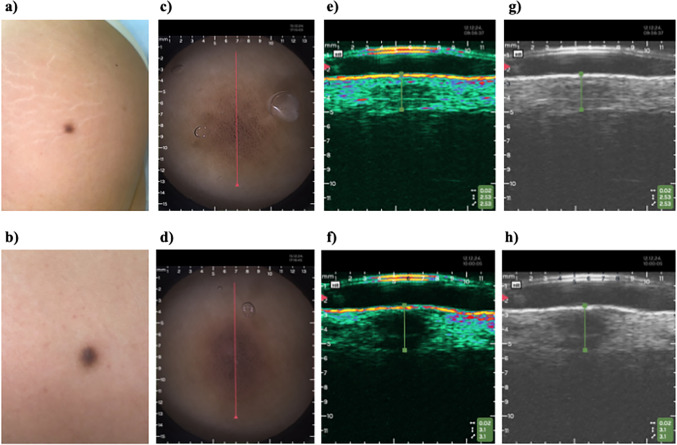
Dermatofibromas in a 23-year-old female patient, Fitzpatrick skin type II. a, b Clinical images showing flat, round, pigmented lesions with irregular borders and a darker, hyperkeratotic central area on the left thigh (a) and right thigh (e). c, d Dermoscopic images of the lesions. e, g Dermoscopy-guided high-frequency ultrasound (DG-HFUS) images of lesion 1, demonstrating a dermal lesion with a depth of 2.4 mm. f, h DG-HFUS image of lesion 2, showing a dermal lesion with a depth of 3.0 mm. Both lesions appear predominantly hypoechoic with small internal hyperechoic areas, show poorly defined margins, and are not sharply demarcated from the surrounding dermal structures. These sonographic features are consistent with dermatofibroma
